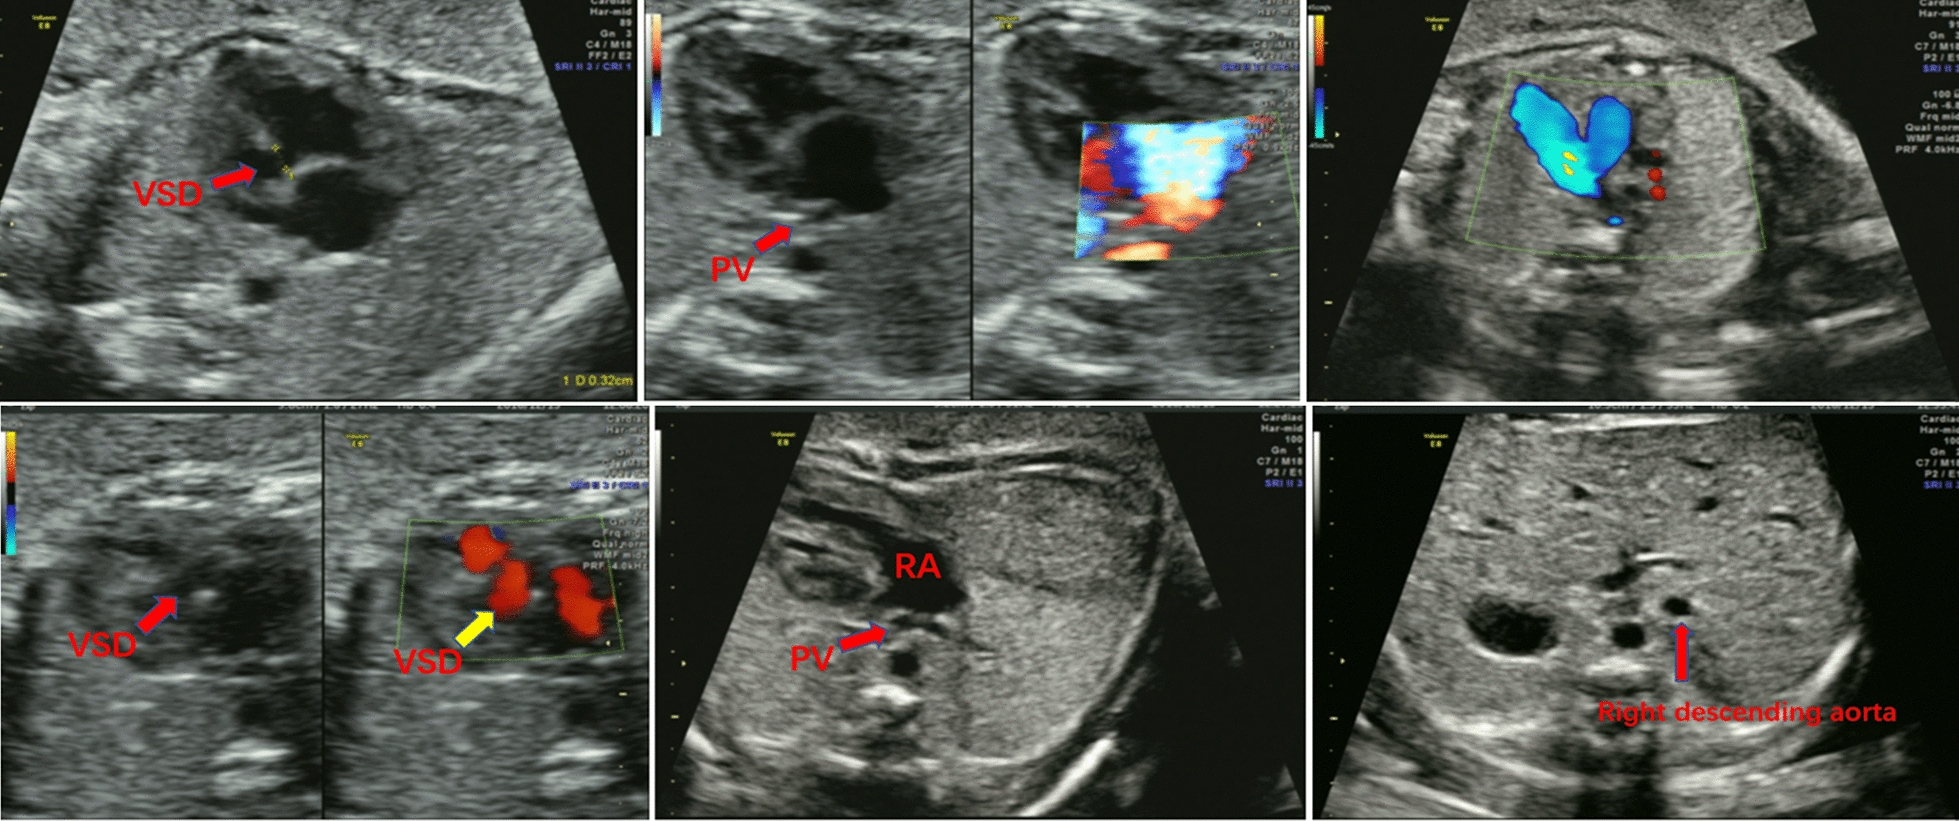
Echocardiography examination of case 2
